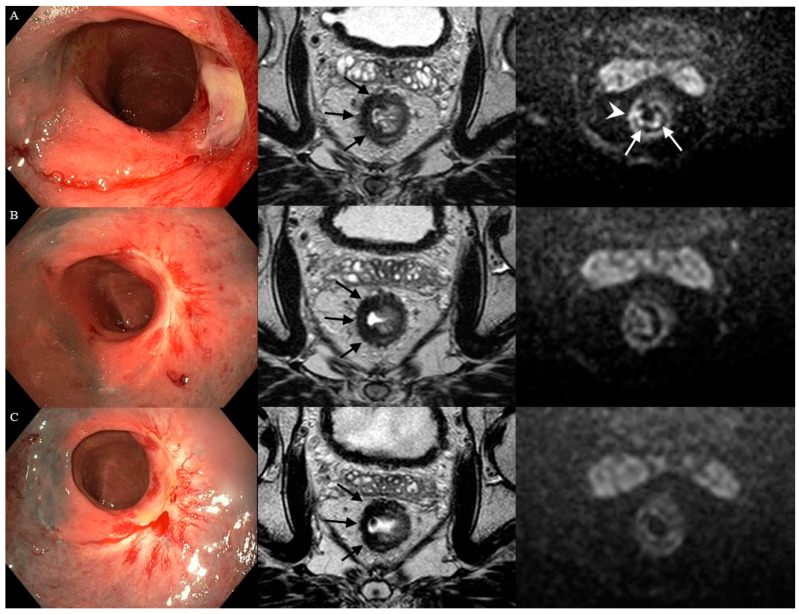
Case of a healing ulcer on endoscopy and the presence of a more diffuse “reactive” mucosal signal on DWI after contact X-ray brachytherapy. Three months following CXB (A), an irregular ulcer on endoscopy, irregular heterogeneous fibrosis (black arrows) on T2W-MRI, and small focal spots of high signal within the fibrosis (white arrowhead) in combination with a diffuse “reactive” mucosal signal on DWI were observed. Later, during follow-up at 7 (B) and 11 (C) months, the ulcer on endoscopy healed into a flat, white scar, the fibrosis on T2W-MRI became more regular and homogeneous, and, on DWI, the small focal spots and the diffuse “reactive” mucosal signal disappeared.
